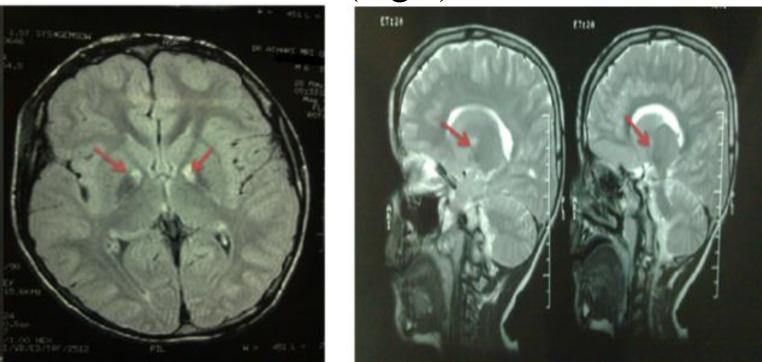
Magnetic resonance imaging (MRI) scans of patient showing bilateral Globus pallidus (GP) abnormality (red arrow). Left: Axial FLAIR brain MRI, shows hyposignality in bilateral GP with a hypersignality in its medial part; Right: Sagittal T2-weighted brain MRI shows hypersignality in medial part of GP.
